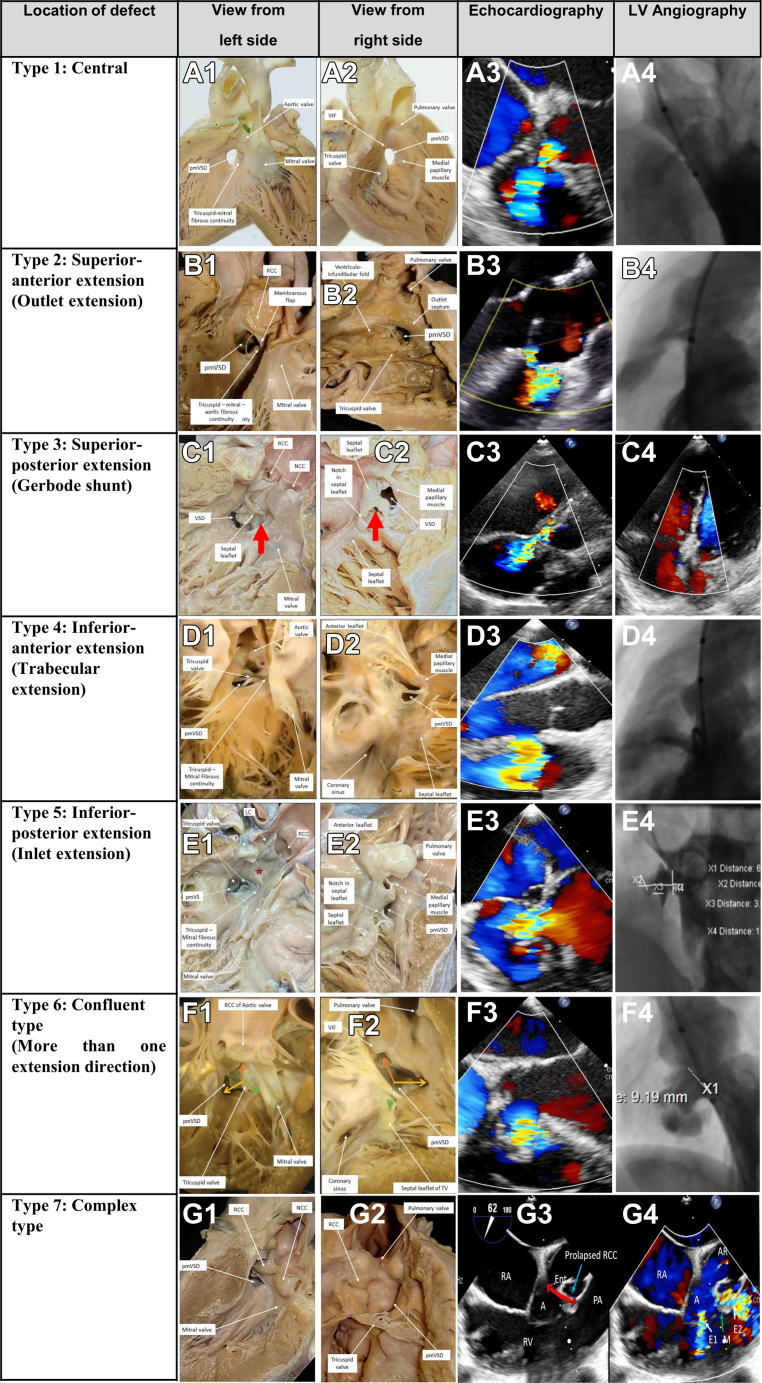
Morphology of pmVSD and Variants Depending on Its Extensions Type 1 includes central perimembranous defect, minimal extension. Type 2 includes outlet pmVSD with antero-superior extension. Type 3 includes pmVSD with posterosuperior extension, causing Gerbode shunt. Type 4 includes trabecular pmVSD with antero-inferior extension. Type 5 includes inlet pmVSD with poster-inferior extension, underlying the septal leaflet of the tricuspid valve. Type 6 includes confluent type pmVSD with 3 directions of extension where an orange arrow indicates antero-superior extension–outlet extension, a blue arrow indicates antero-inferior extension–trabecular extension, and a green arrow indicates posteroinferior extension–inlet extension. Type 7 is a complex type comprising membranous septum defect and outlet septum defect. Red stars (in A1, D1, E1) indicate muscular rim between the defect and the aortic valve. In outlet pmVSD (B1), a thin membranous flap (membranous remnant) is suspended between the superior defect border and the aortic valve. Red arrows (in C1, C2) indicate the sites of the Gerbode shunt owing to cleft in the septal leaflet. In complex type (G3, G4), a transesophageal echocardiography modified short-axis view shows the entrance extending beneath the noncoronary cusp (NCC) and the right coronary cusp (RCC), with the RCC prolapse partially covering the defect (blue arrow). On the RV side, the defect divides into 2 exit points (E1/E2): E1 located posteroinferiorly associated with aneurysm (A), and E2 located antero-superiorly just beneath the pulmonary valve and the pulmonary artery (PA). A muscular septal insertion in G4 (M, green arrow) is visible between the 2 exit points. AR = aortic regurgitation; Ent = ; LC = ; M = ; pmVS = ; TV = tricuspid valve; RA = right atrium; VIF = ventricular infundibular fold; other abbreviations as in Figure 1.
